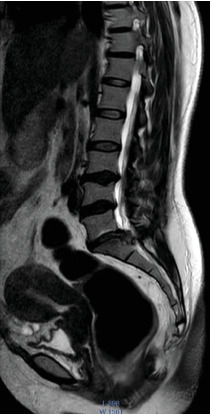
MRI showing diffuse disc bulge at multiple lumbar levels, caudal migration of extruded disc at S1 vertebral level causing compression of adjacent nerve roots with severe spinal canal stenosis.
